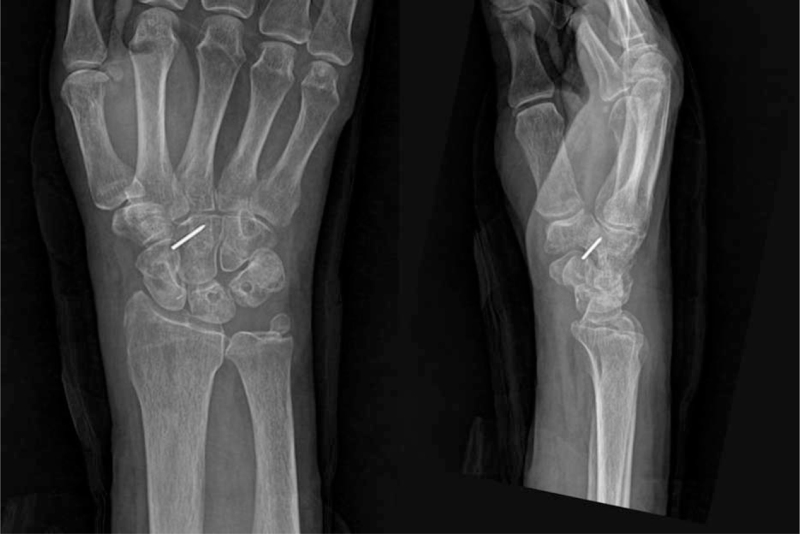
Posteroanterior and lateral radiographs at 6 months after surgery. One K-wire broke and a part of wire remained in the bone. The radiographs showed good alignment of the carpal bones and no arthritic changes.
